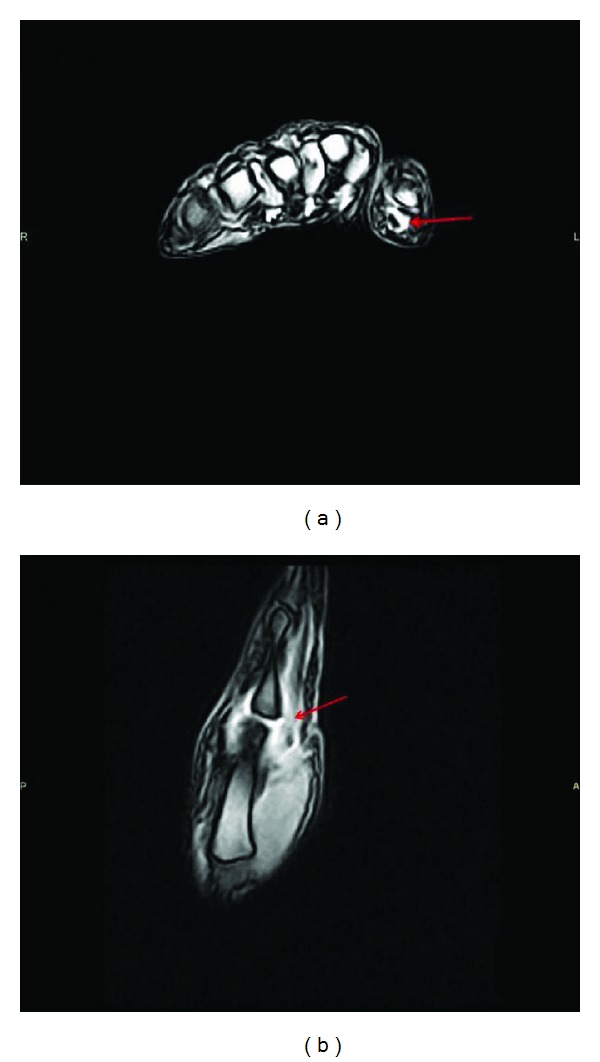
(a) T2 weighted MRI of the left hand shows a fluid distension of the sheath of the flexor tendons of the thumb. (b) T2 weighted MRI of the left hand shows fluid distension of the joint capsule of the 1st metacarpophalangeal.
